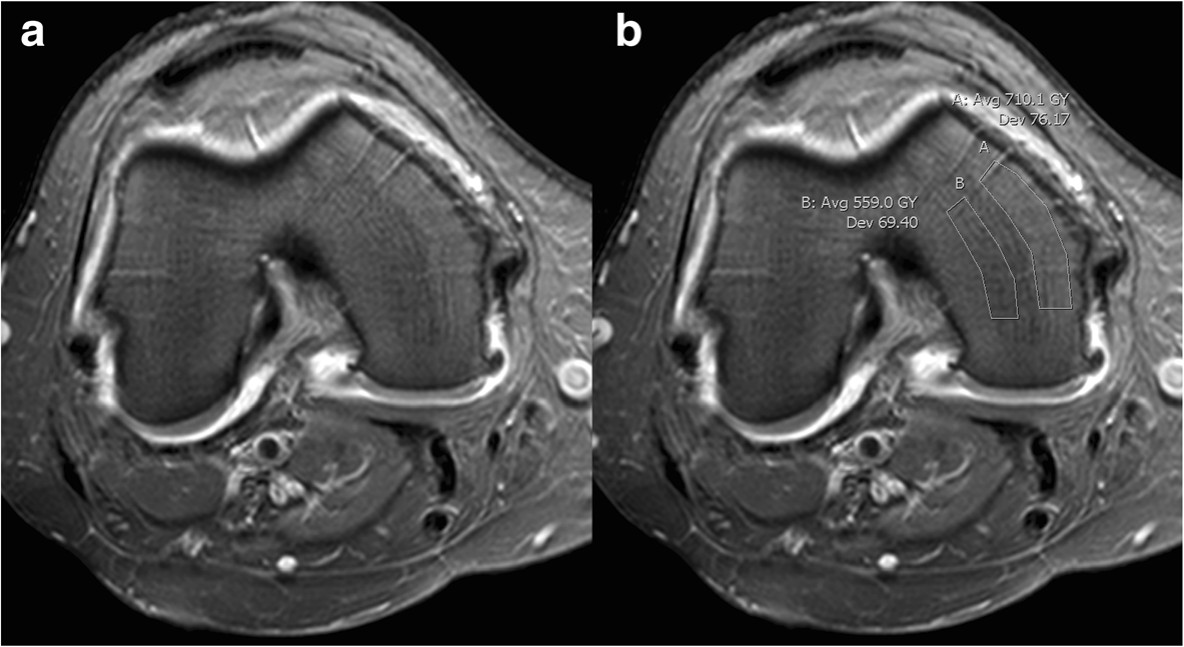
Trans-axial post-contrast T1 FS MR images of the femoral condyles. Two identical images without (a) and with (b) calculations of Gray (GY), performed in Impax. The difference in signal intensity is barely visible in image (a) between the medial and central (lateral) part of the medial femoral condyle. Yet, there is a difference in GY between the areas of 151.1 GY, meaning the average GY in the central portion is ~ 21% lower than in the medial portion. Also note the blood vessels perforating the condyles from the periphery
